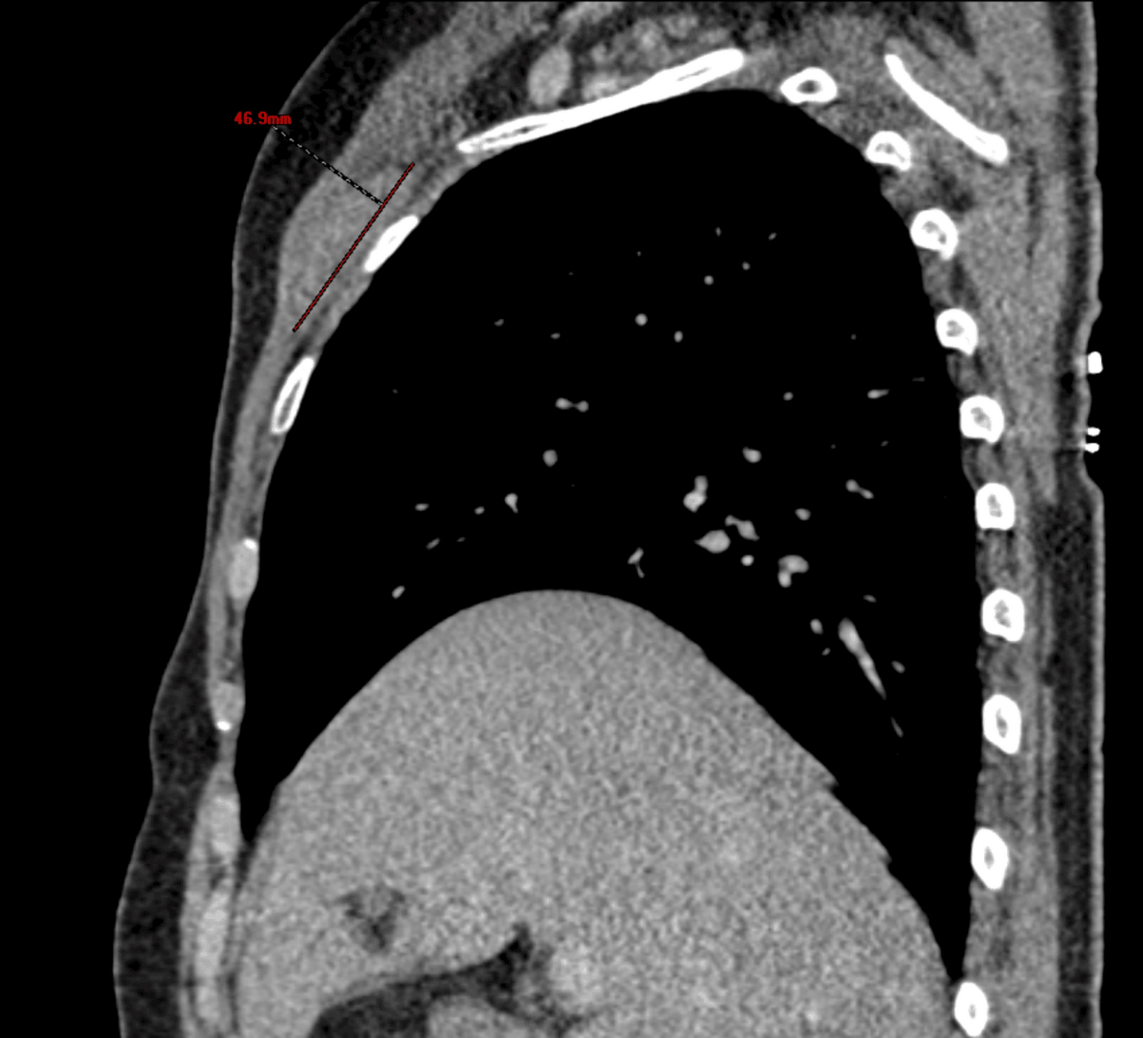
Sagittal contrast-enhanced CT scan of the chest showing a 4.7 cm subfascial soft-tissue mass (measured in red) located along the right anterior chest wall, involving the pectoralis minor muscle.
